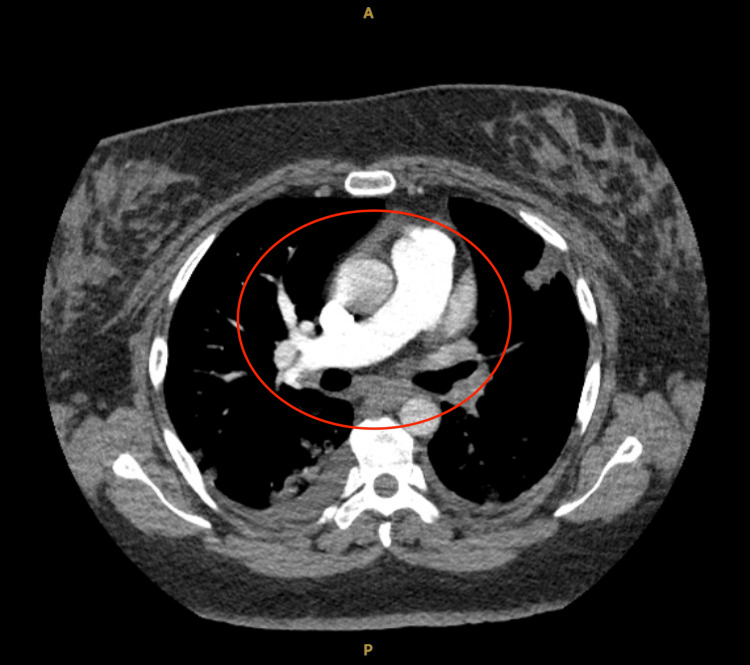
CT angiogram of the chest (axial) showing extensive involvement (noted within the red circle) of the left main pulmonary artery with complete opacification of all distal arterial segments on the left side, including interlobar and subsegmental arteries.
